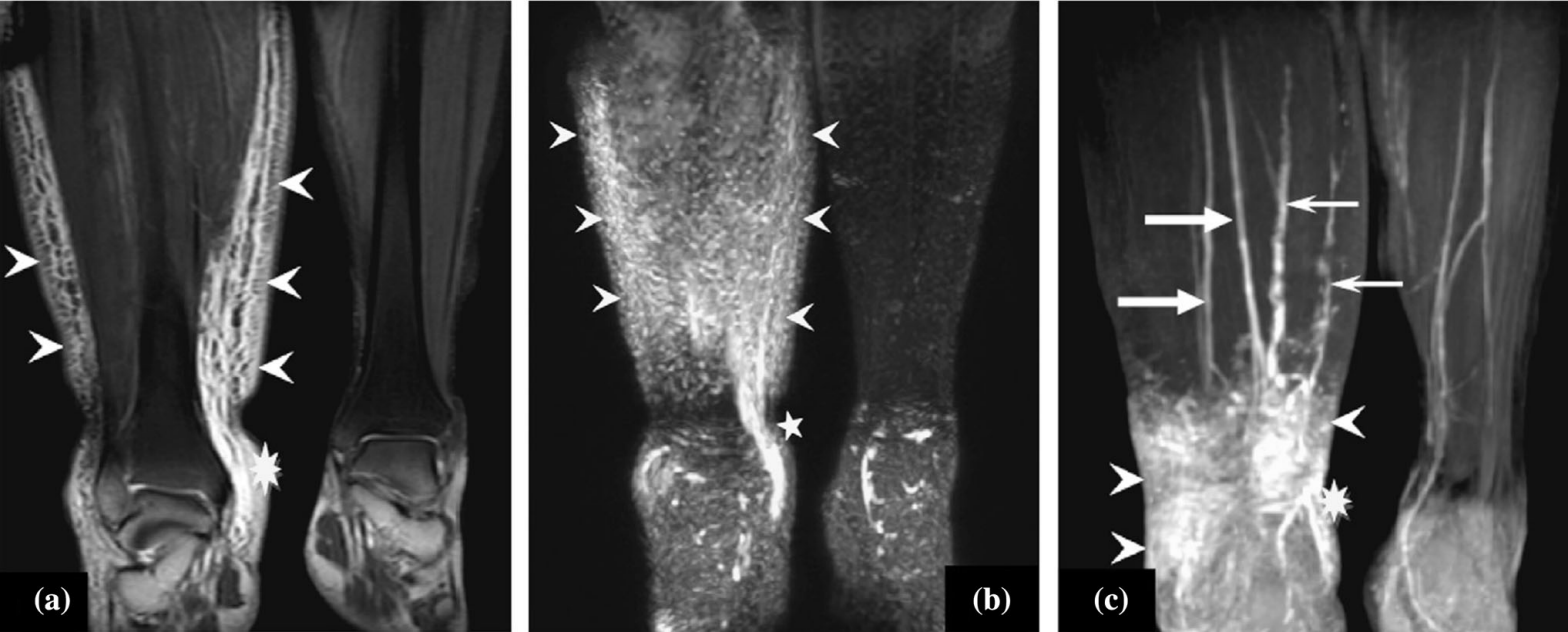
a Coronal T2-weighted 2D-TSE image with fat suppression shows an extensive reticular pattern of dilated lymphatic vessels, indicating neovascularization due to obstruction in the right lower leg (arrowheads). b Frontal 3D heavy T2-weighted MIP image demonstrates the same changes in the right lower leg (arrowheads). c Frontal 3D spoiled gradient-echo T1-weighted MRL MIP image obtained 35 min after Gd-BOPTA injection. Two slightly enlarged lymphatic vessels are visualized in the affected right lower leg (small arrows). The concomitantly enhanced veins (large arrows) show lower signal intensity. Furthermore, areas of accumulated lymph fluid are detected in the three modalities image (asterisk). No lymphedema is seen in the left lower leg. *2D-TSE* two-dimensional turbo spin-echo, *3D* three-dimensional, *MRL* magnetic resonance lymphography. Reprinted from Lu et al.^162^ with permission from Elsevier
